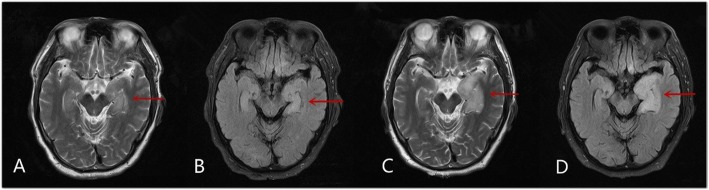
Magnetic resonance imaging of Case eight. a-b Magnetic resonance imaging (FLAIR) shows abnormal signals in the left hippocampus, as indicated by the red arrow. c-d One month later, the clinical symptoms of the patient were aggravated, and repeated magnetic resonance imaging shows an enlargement of the lesion
